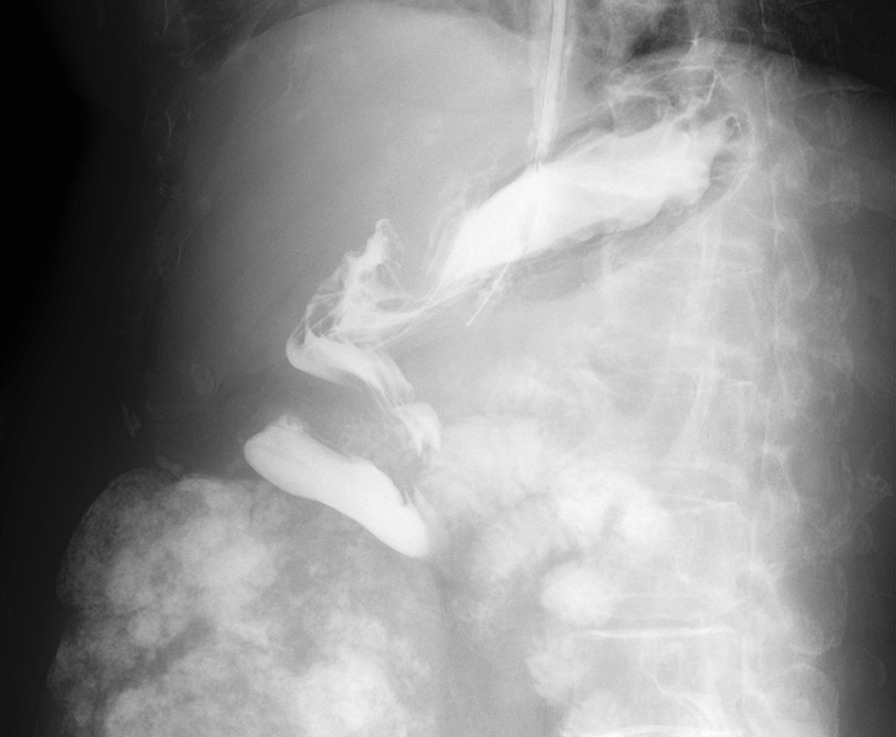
Upper gastrointestinal tract radiography reveals the rotation of the gastric body around the organoaxis
